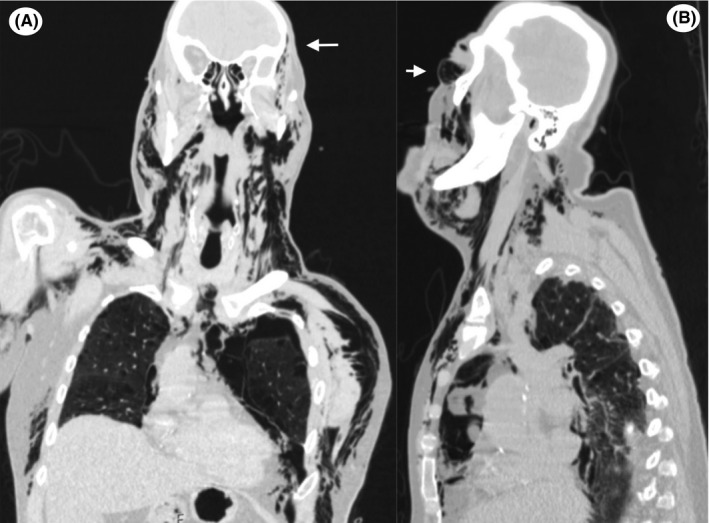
A, Computed tomography scan demonstrating the extension of the emphysema up to the temporal subcutaneous tissue. B, Computed tomography (orbital view)
